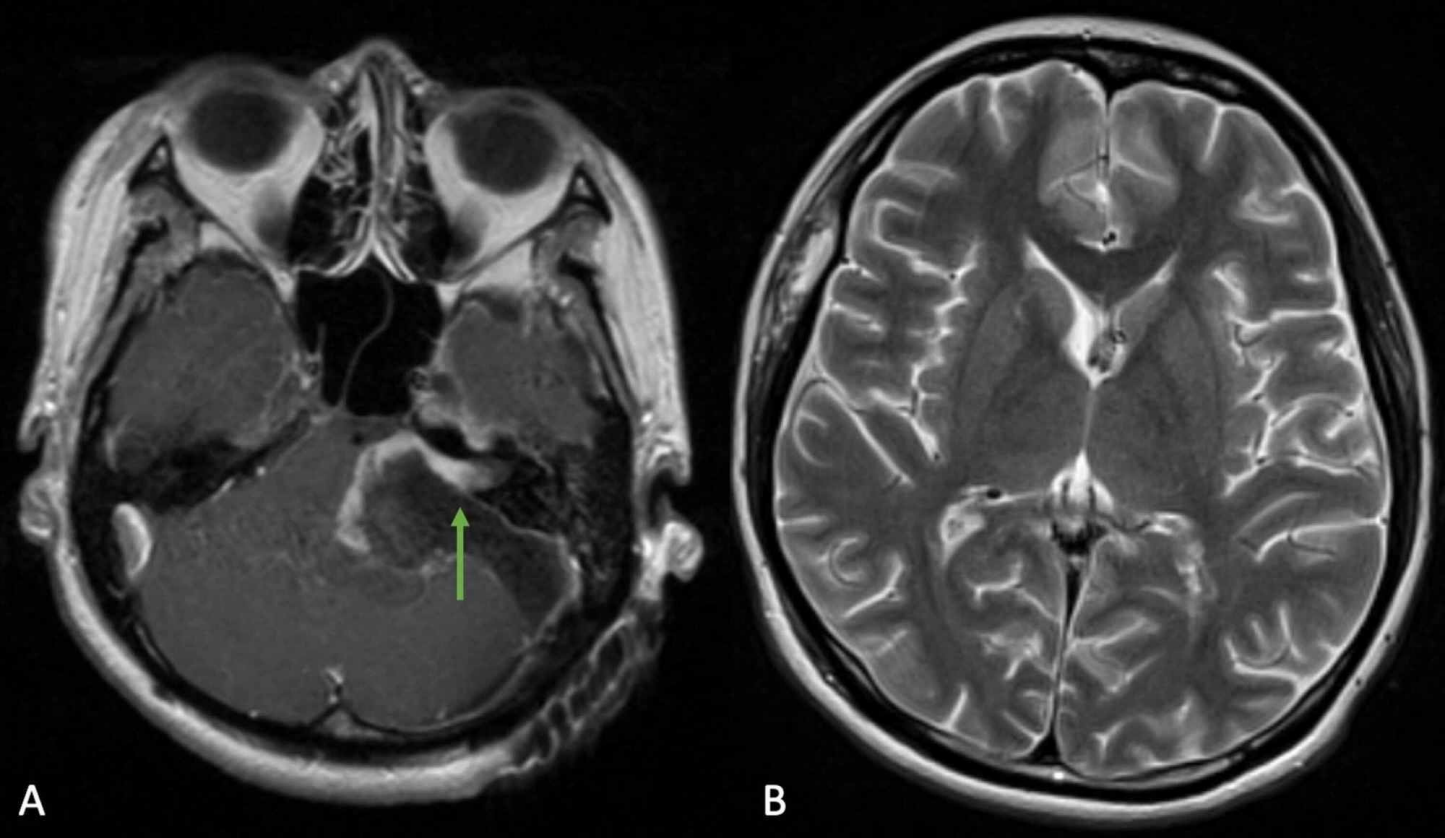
Postoperative MRI after retrosigmoid craniectomy for left-sided vestibular schwannoma resection which resulted in near total improvement of right V2 sensory dysfunction. (A) Axial T1-contrasted MRI shows resection of vestibular schwannoma with residual tumor capsule left adherent to facial nerve at the porus acousticus and improvement of rightward brainstem deviation (green arrow). (B) Axial T2 MRI shows resolution of hydrocephalus.
